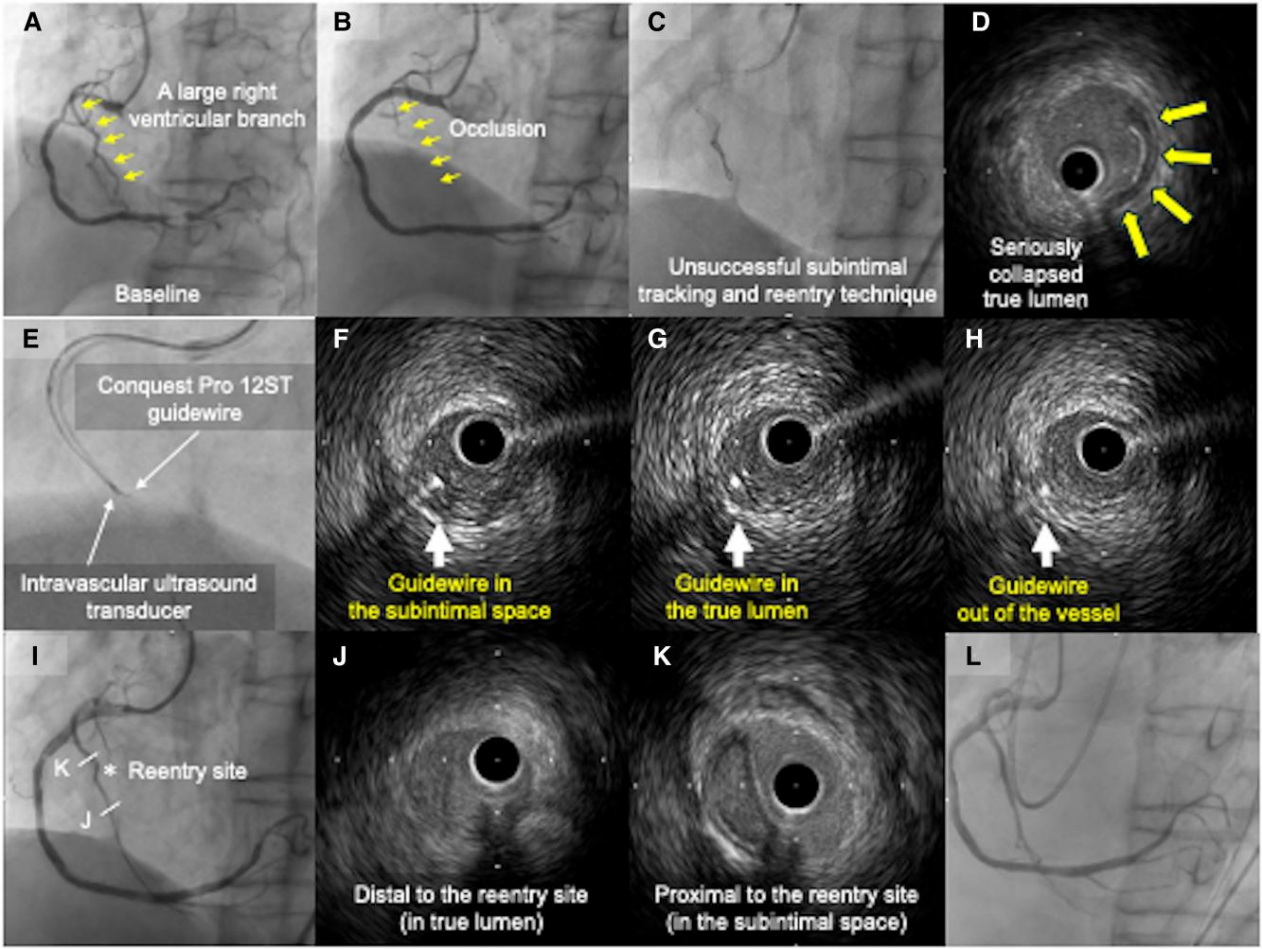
Images during the tip-detection antegrade dissection and re-entry into a seriously collapsed true lumen. (*A*) Baseline angiography. (*B*) A large right ventricular branch had occluded (small arrows). (*C*) Bailout sub-intimal tracking and re-entry technique was unsuccessful. (*D*) The true lumen became seriously collapsed. (*E–H*) Images during the TDADR. (*I*) The asterisk is the re-entry site of the TDADR. (*J*) The intravascular ultrasound image, distal to the re-entry site, shows the true lumen route and (*K*) the sub-intimal route proximal to it. (*L*) The angiography on the following day.
